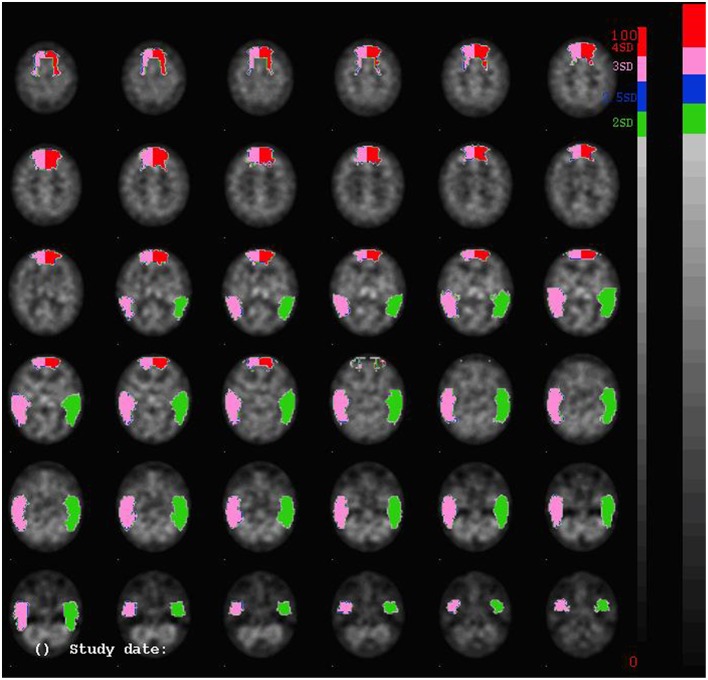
SPECT-findings demonstrating a significant decrease of perfusion bilaterally in the prefrontal and medial frontal regions, as well as in the lateral temporal regions.
